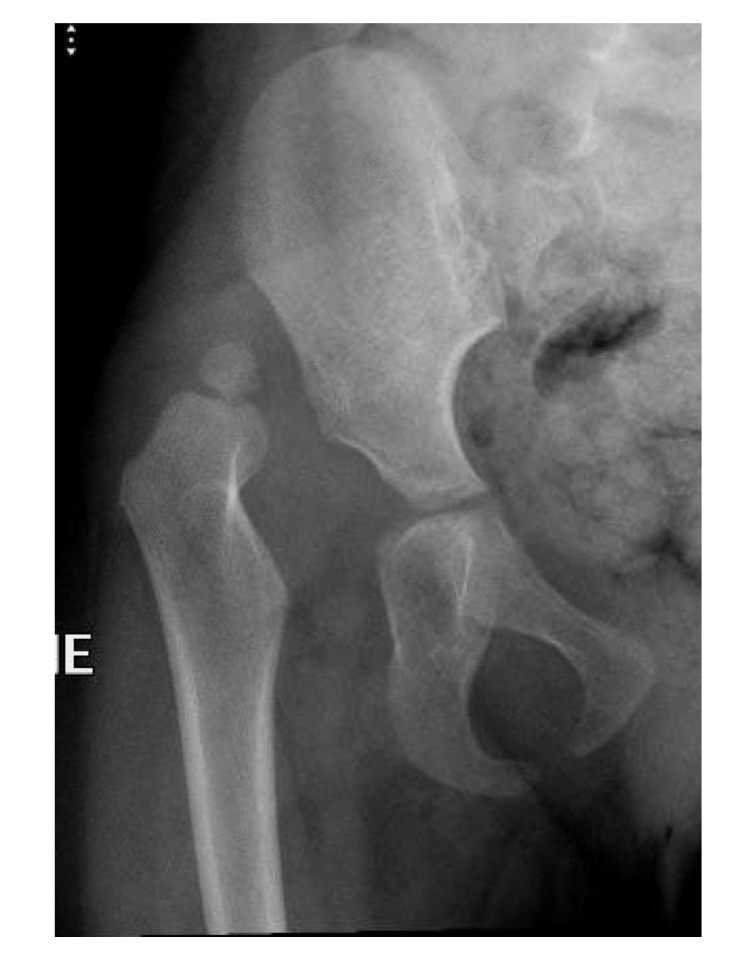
Plain radiograph of a girl at age 2 years and 9 months.
